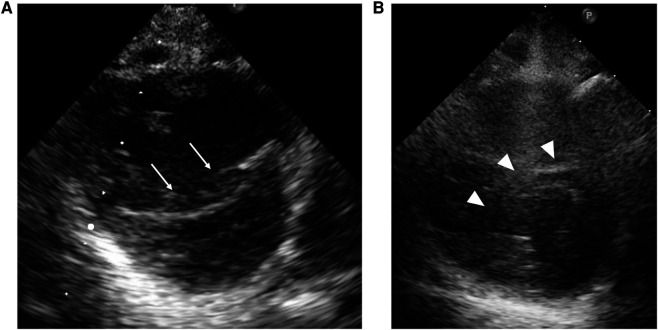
Case 2: echocardiography in the parasternal short-axis view. Echocardiography at the beginning of epoprostenol infusion (A) shows that the inverted interventricular septum (white arrow) compresses the left ventricle. At the time of epoprostenol infusion above 100 ng/kg/min (B), the interventricular septal shift (white arrowhead) and LV compression ameliorated.
